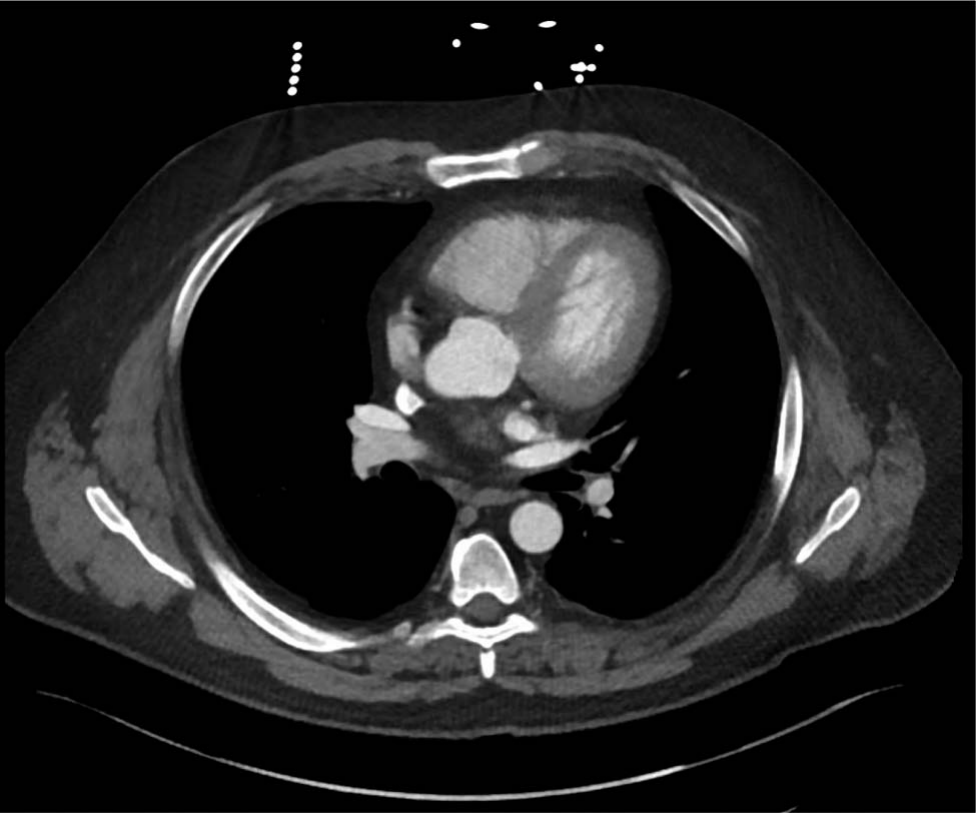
Computed tomography angiography of the chest showing no evidence of aortic dissection.
